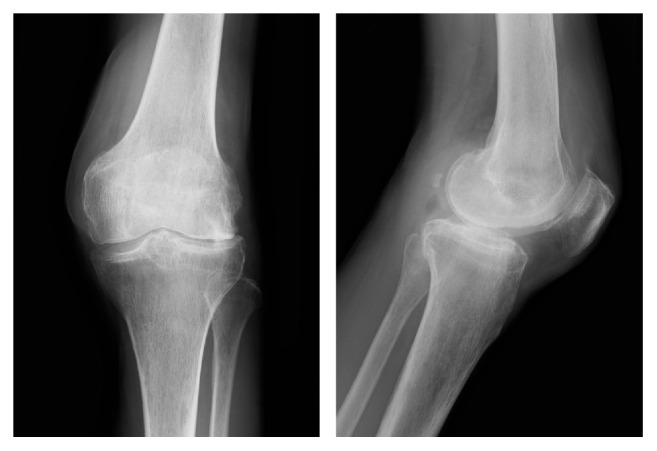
Left knee radiography at 30 months after the onset reveals progressive narrowing of the lateral joint space and osteophyte formation.
